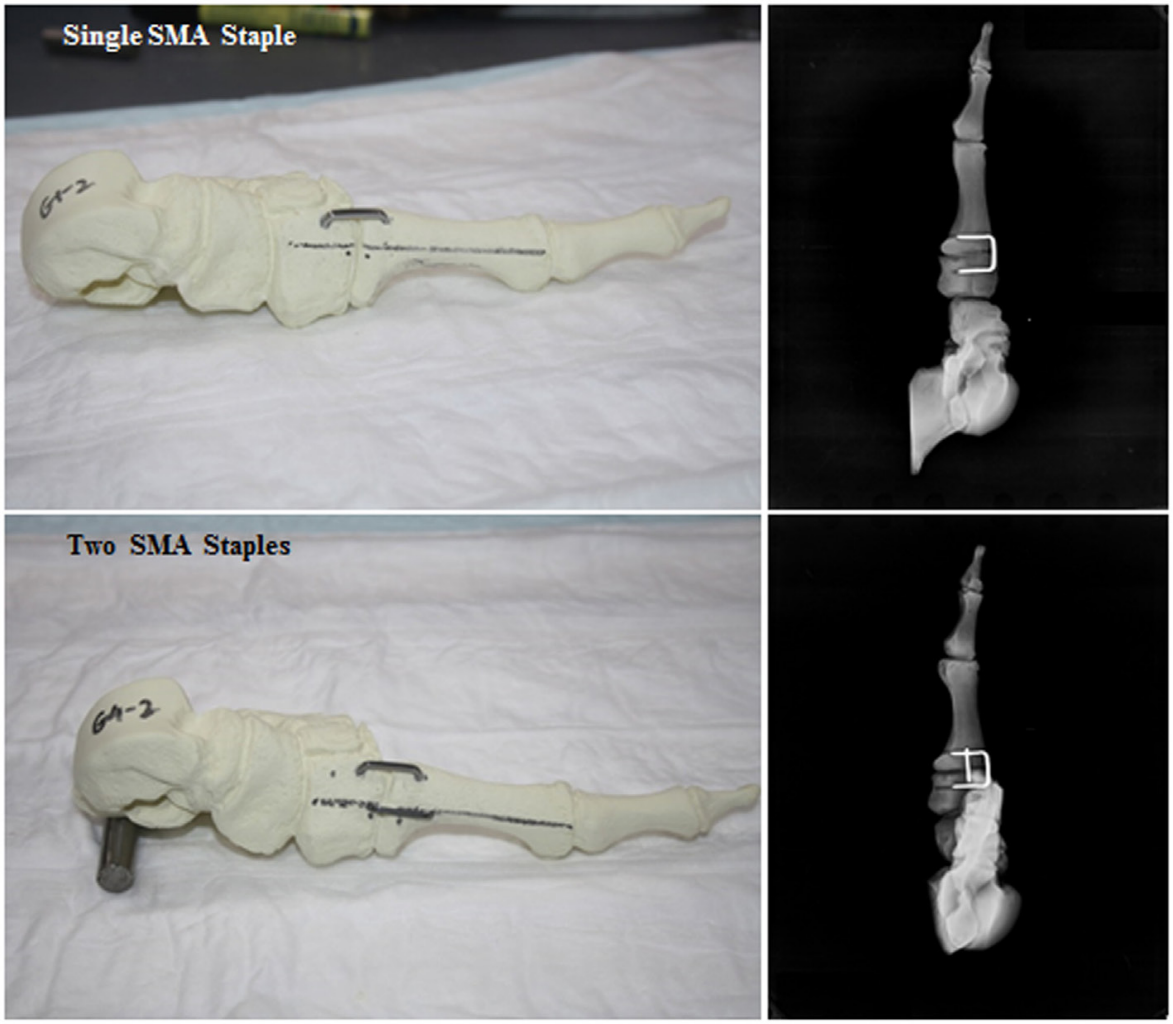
Digital photographs and faxitron radiographs showing the assembled single SMA staple and double SMA staple constructs.
